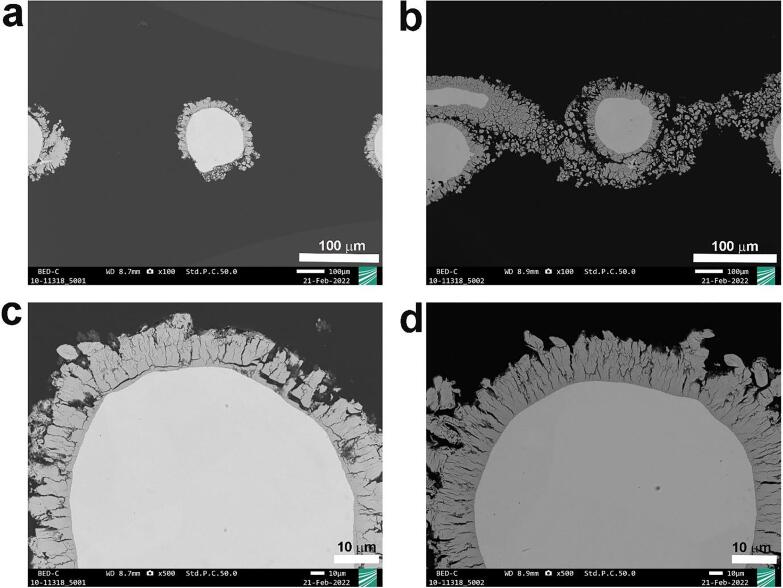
Cross-section SEM images of Raney-Ni at different magnifications: a) and c) without ultrasonication (*silent* conditions), b) and d) after ultrasonication for 15 min in 30 wt.-% aqueous KOH solution at *T* = 25 °C.
